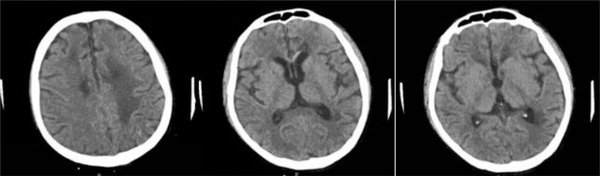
CT images of the patient's brain 3 months after transplantation. The nodules in the brain parenchyma became significantly smaller than before
